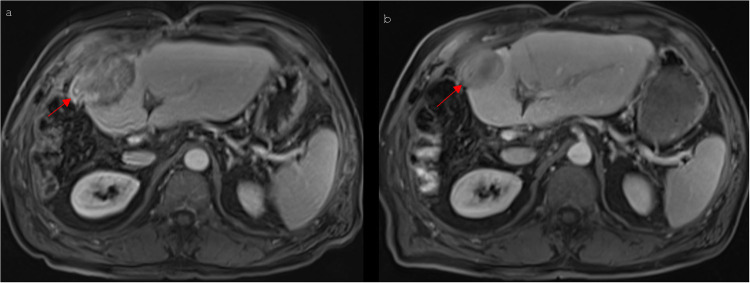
Signal intensity of the ablation zone over time. Different MR images obtained in the center (a) and in the peripheral zone (b) of the ablation area (c.e.^1^: contrast-enhanced; P.V.^2^: portal venous). Significant differences during the follow-up are highlighted (*).
